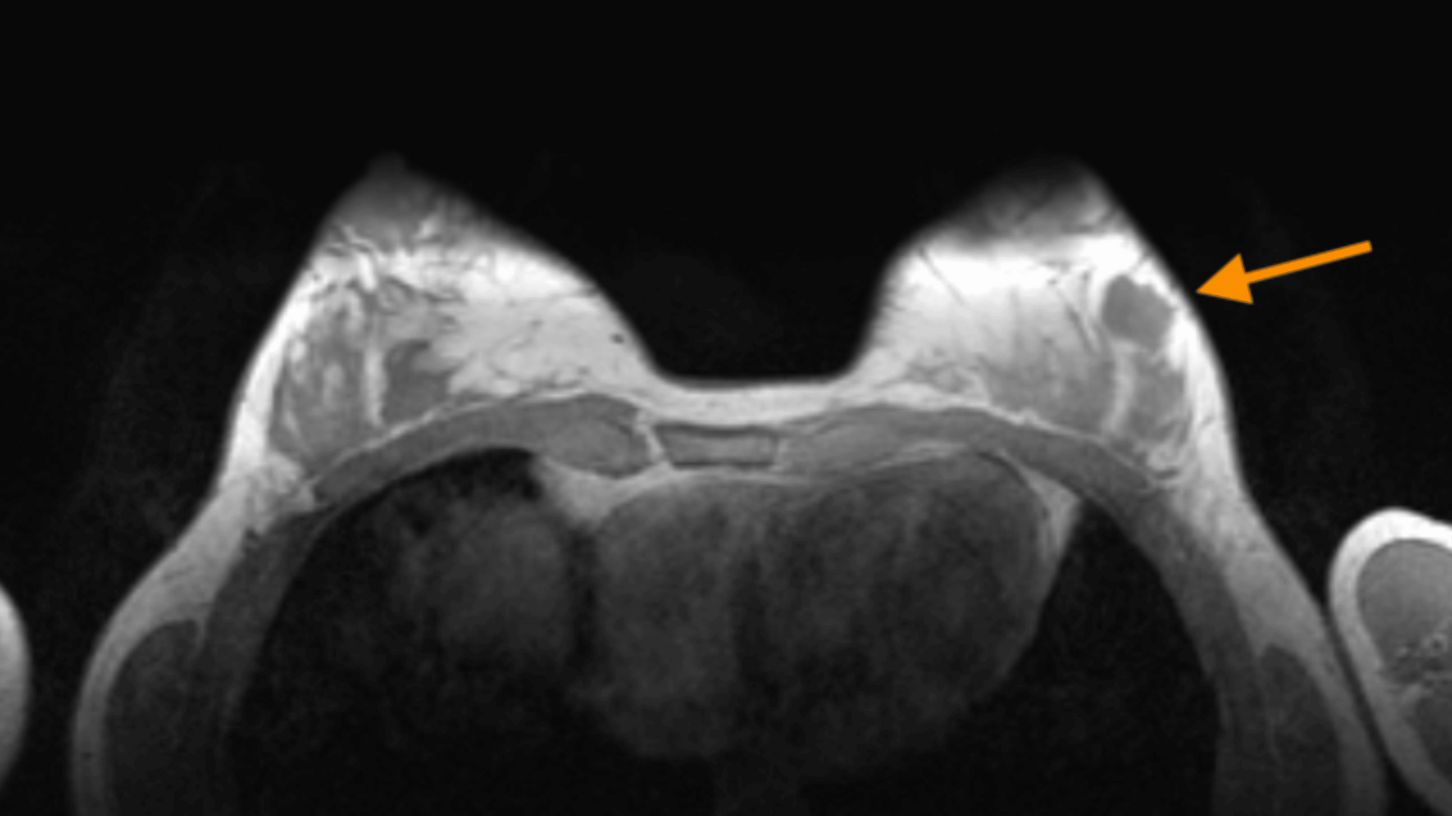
The MRI (T1WI) showed a solid lesion with lobular margins located at the four o’clock position of the left breast (arrow) showing hypointensity on T1WIs. MRI: magnetic resonance imaging; T1WI: T1-weighted images
